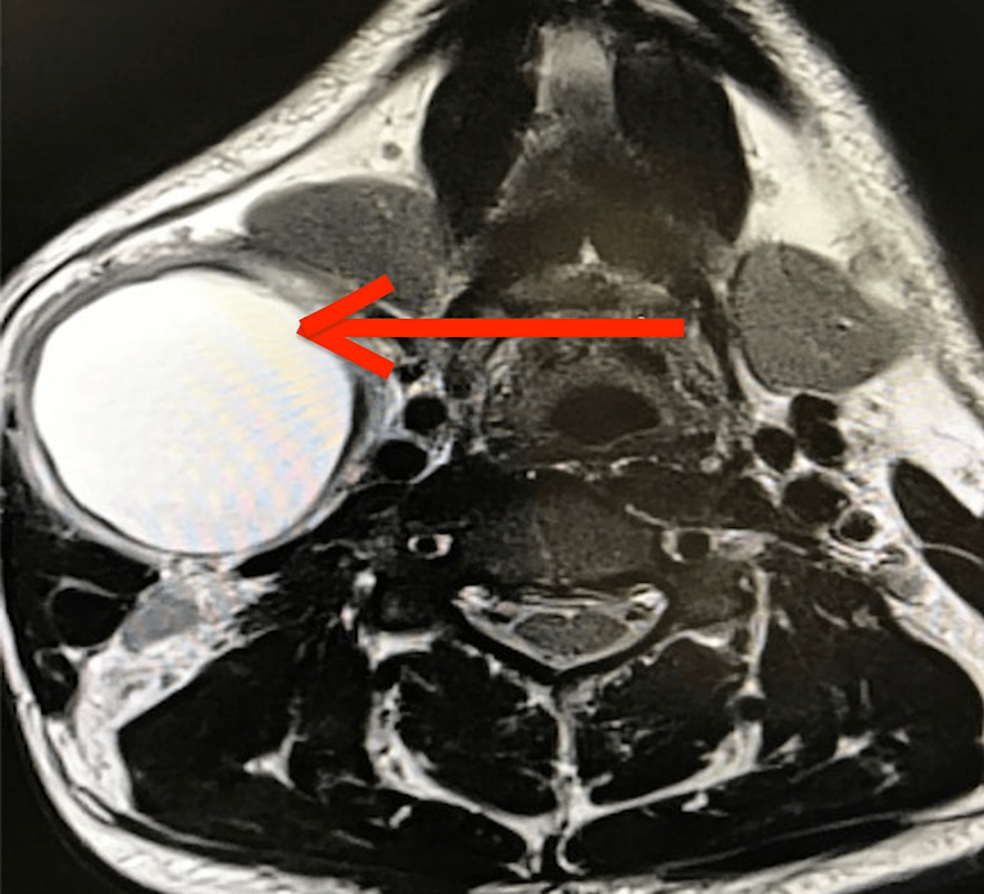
T2 cervico-facial MRI in axial section found a cystic mass under the right angulo-mandibular with a thick wall in its upper part of the same dimensions associated with gonglions in the territories of IIa and IIb suggesting a cyst of the second branchial cleft (red arrow).
